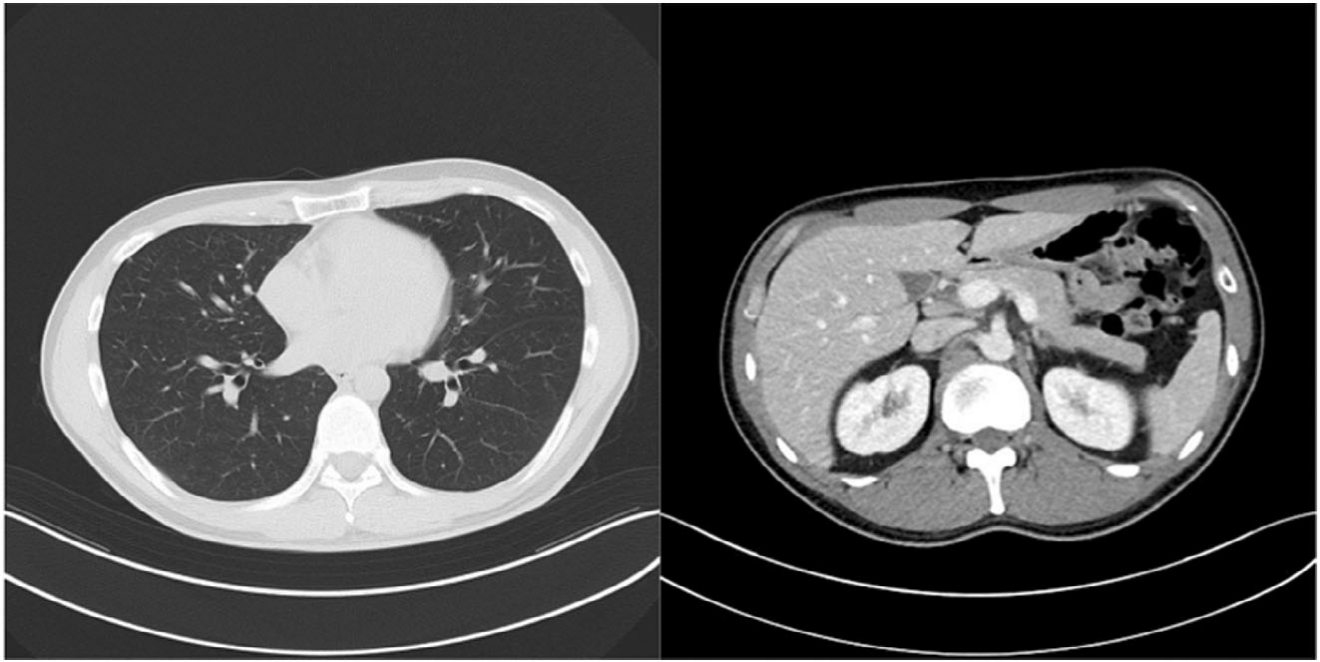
CT imaging showed CR at cycle 4 and subsequent evaluations.
